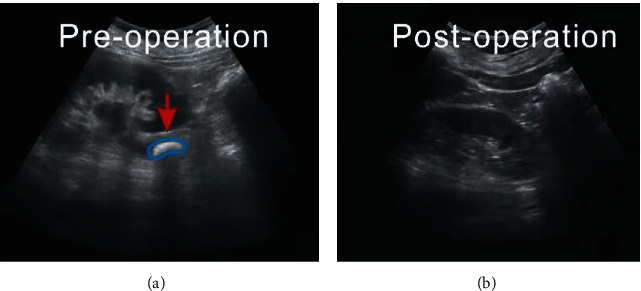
Ultrasound scan image of ureteral stones in subject 1 ((a) original scan image and (b) postoperative scan image). The arrow points to the location of the ureteral stones. Note that the stones were located much easier by the radiologists after image quality enhancement. This demonstrates that the medical image deblur and denoising algorithms are useful and have strong clinical applicability in accurate medical image diagnosis.
